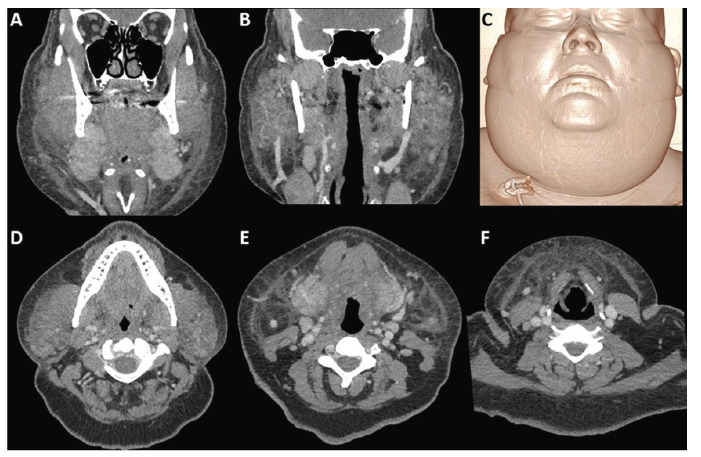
Coronal (A-B) and axial (D-F) CT neck with contrast. 3D volume rendering reformatting (C). Diffuse fatty stranding of the lateral and anterior neck, with thickening of the platysma muscles. The process extended to the parapharyngeal, parotid, and submandibular spaces, with reactive sialadenitis. Multiple sub-centimeter lymph nodes and mucosal/submucosal edema of the oropharynx were observed. No drainable/organized collections identified.
